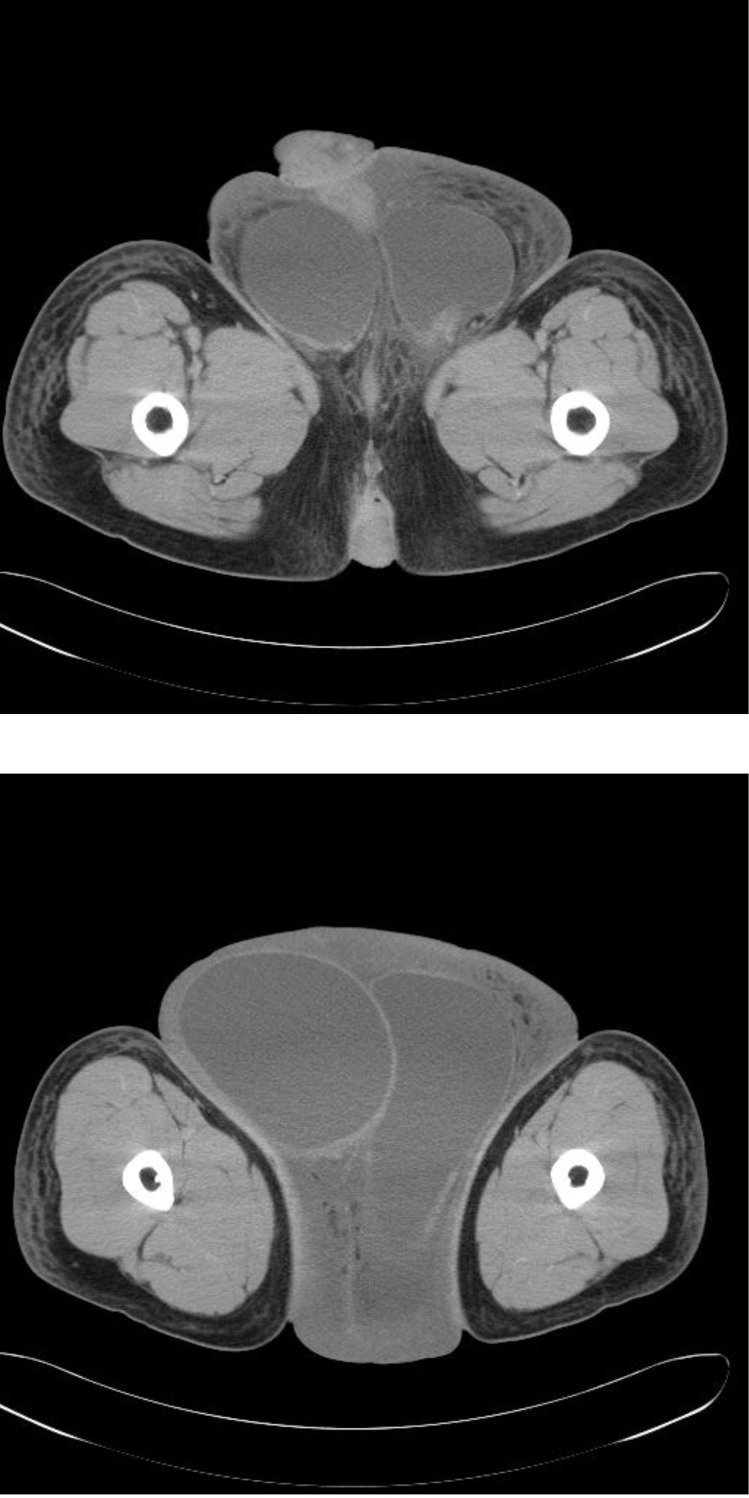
Computed tomography scan: scrotum enlargement of the scrotum
